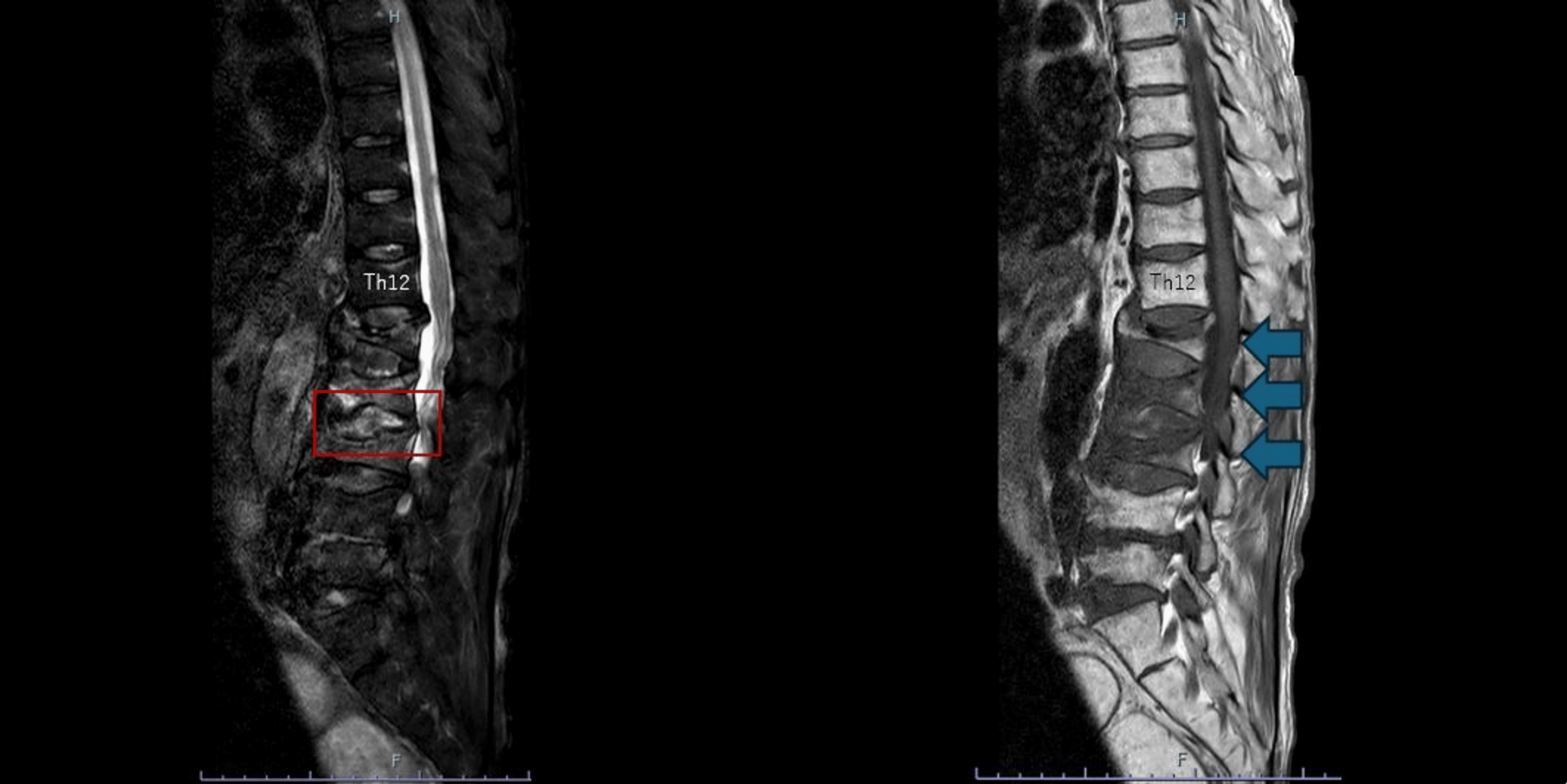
Lumbar spine MRI showing T2-weighted fat-suppressed images (left) and T1-weighted images (right) On T2-weighted fat-suppressed images, the L2/3 intervertebral disc demonstrates high signal intensity. On T1-weighted images, decreased signal intensity of the bone marrow from L1 to L3 is observed, consistent with acute compression fractures. MRI: magnetic resonance imaging
